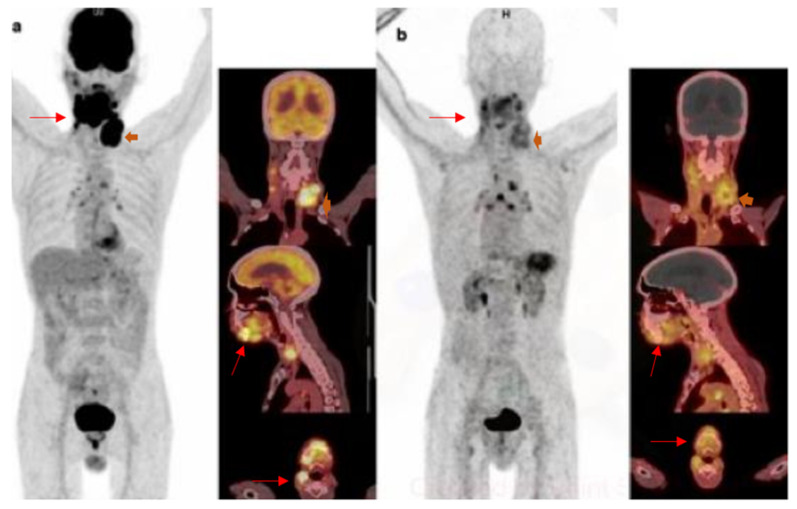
^18^F-FDG and ^68^Ga-Pentixafor images of a 60-year-old female with squamous cell carcinoma of the oral cavity with intense uptake in the primary lesion and left cervical lymph node metastasis on ^18^F-FDG (a). There was mild tracer accumulation on the ^68^Ga-Pentixafor PET (b). Interestingly, the inflammatory mediastinal lymph nodes appear more intense on the ^68^Ga-Pentixafor PET.
